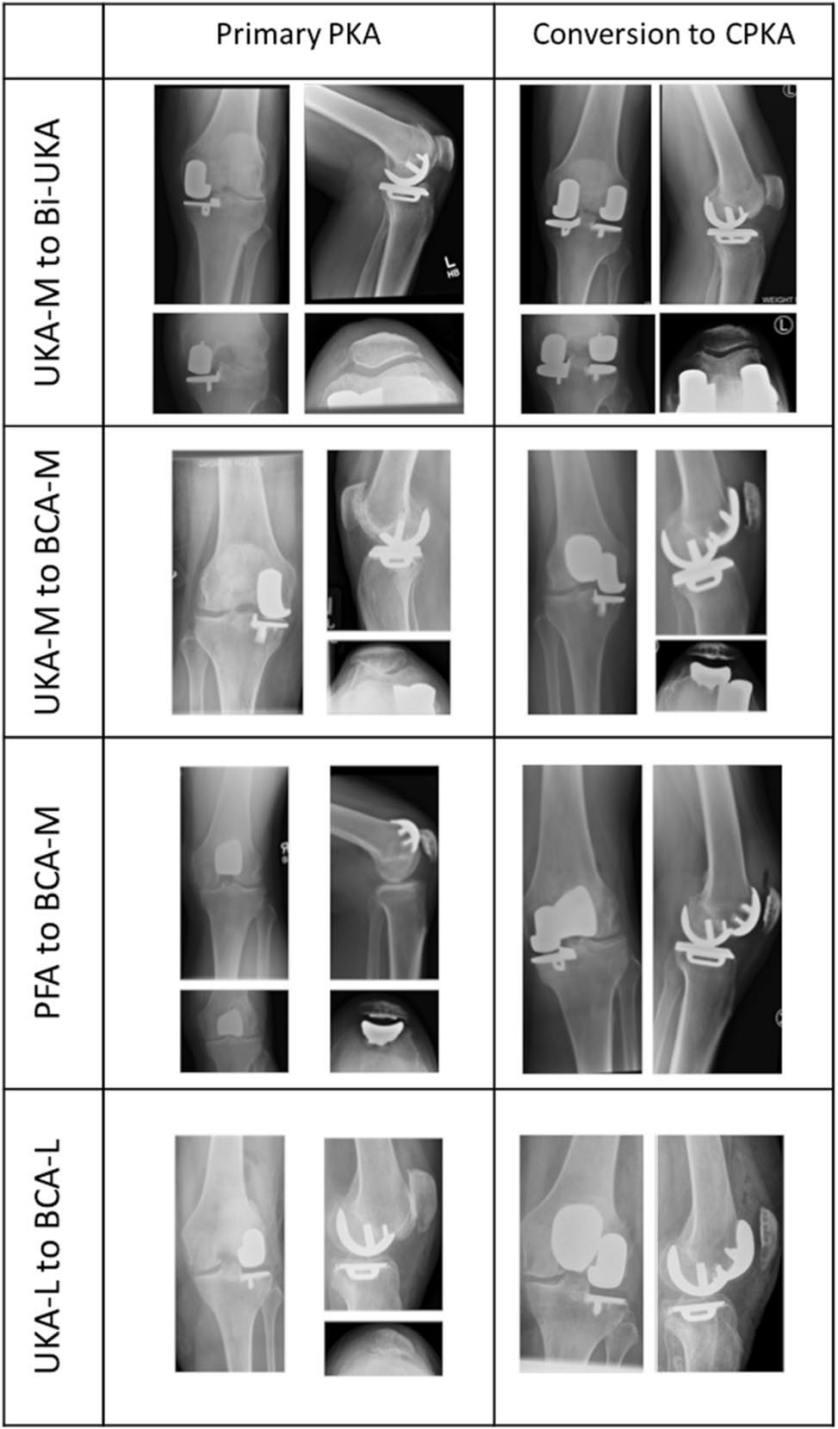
Radiographic examples of partial knee arthroplasty (PKA) procedures revised to combined partial knee arthroplasty (CPKA) for native compartment degeneration, using a compartmental approach. Medial unicompartmental arthroplasty (UKA-M), lateral unicompartmental arthroplasty (UKA-L), patellofemoral arthroplasty (PFA), medial bicompartmental arthroplasty (BCA-M), lateral bicompartmental arthroplasty (BCA-L), bi-unicondylar arthroplasty (Bi-UKA)
